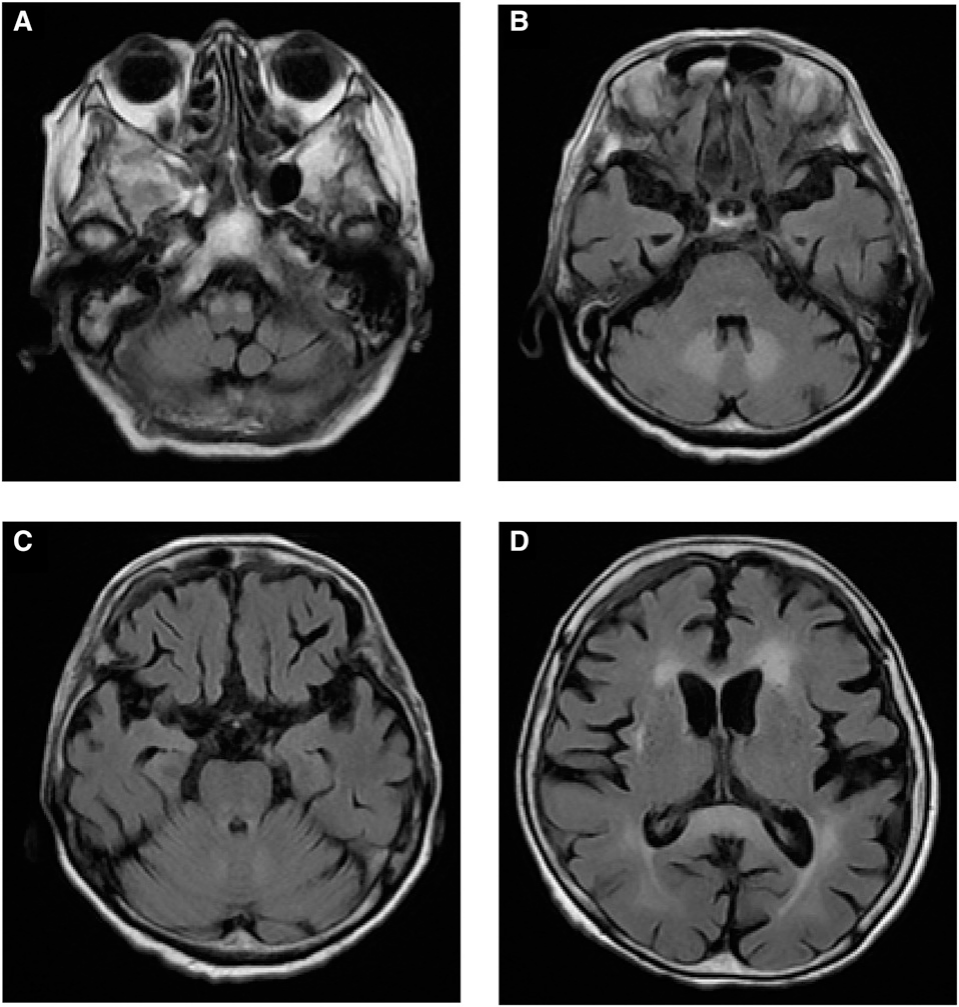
Fluid-attenuated inversion recovery magnetic resonance imaging after the start of metronidazole treatment. The scans after the start of metronidazole treatment reveal bilaterally symmetrical high signals in the (A) bulb olivary nucleus, (B) cerebellar dentate nucleus, (C) pons, and (D) splenium of the corpus callosum.
